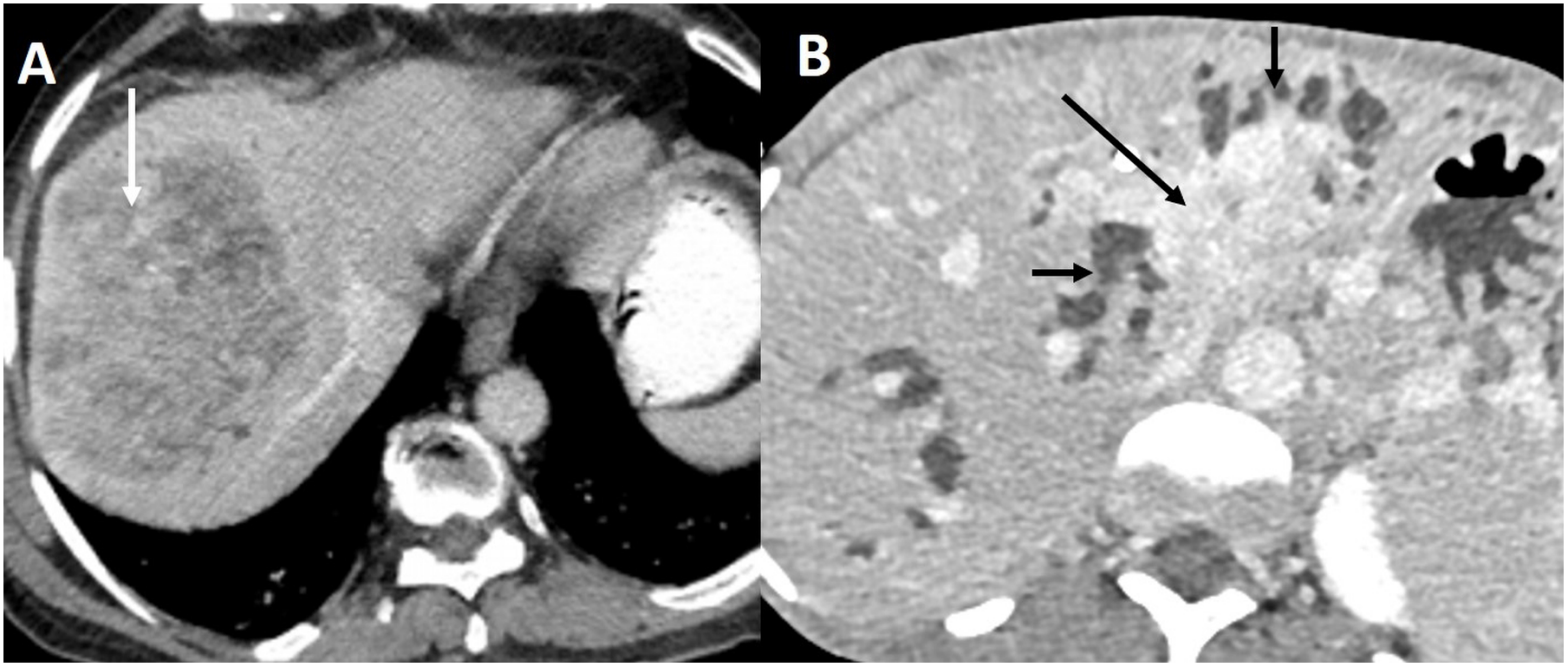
Imaging appearance of small and large duct intrahepatic cholangiocarcinoma. (A) Contrast-enhanced CT in the portal venous phase shows small duct ICC as a heterogeneously enhancing mass (arrow) in the periphery of the liver without bile duct dilatation. (B) Large duct ICC appears as an enhancing mass (arrow) infiltrating the left sided bile ducts causing biliary dilatation (short arrows) in the delayed phase CT.
